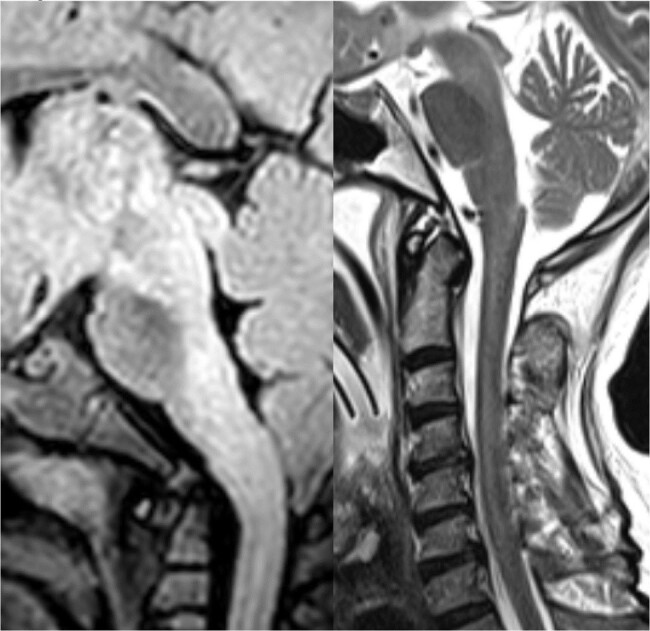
FLAIR/T2 images showing hyperintensities in dorsal brainstem, cervical spinal cord
